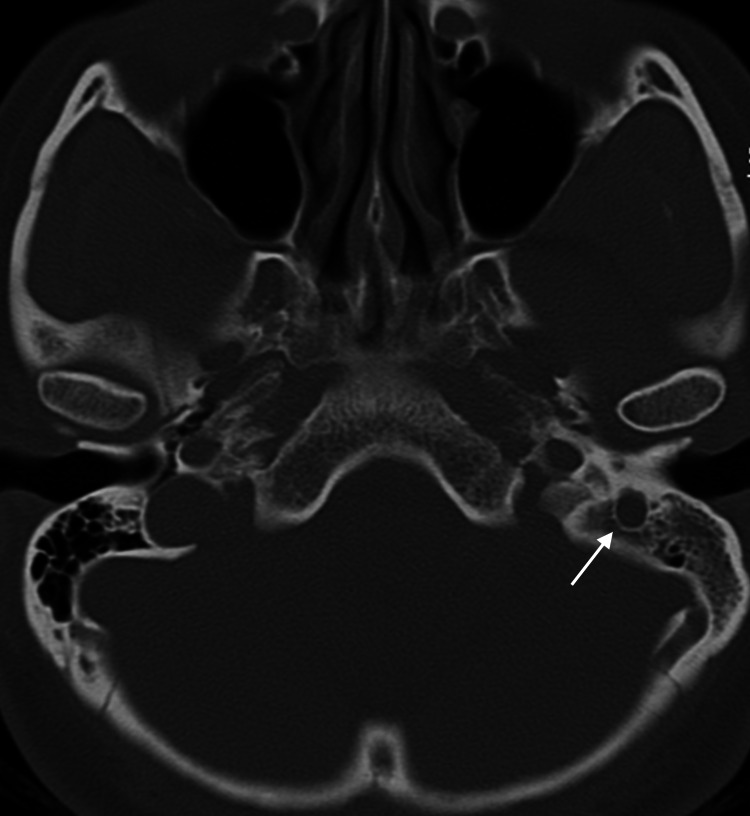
An axial view of high-resolution CT of the temporal bone. Arrow shows a widening of the stylomastoid foramen likely due to enlargement of the facial nerve.
